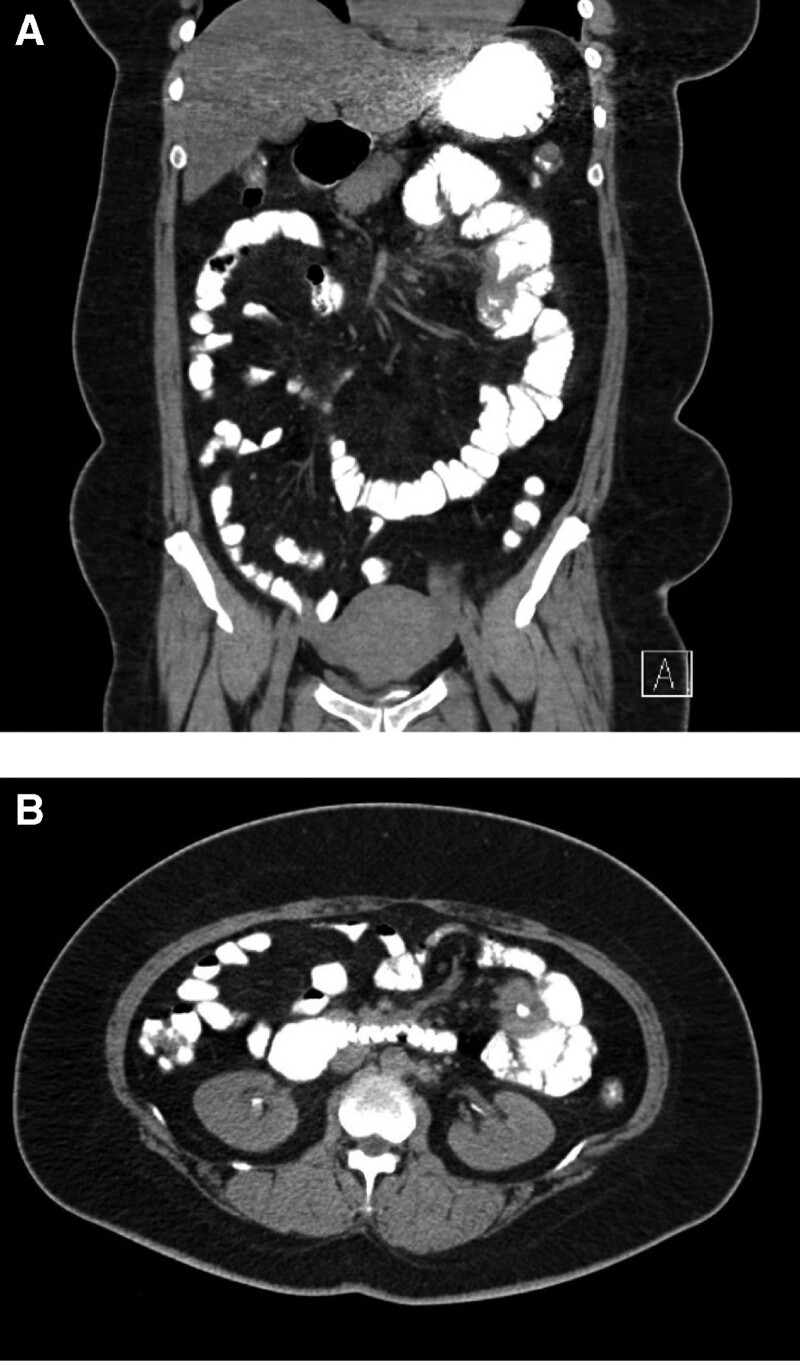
Pre-operative abdominal CT scan. CT = computed tomography.
